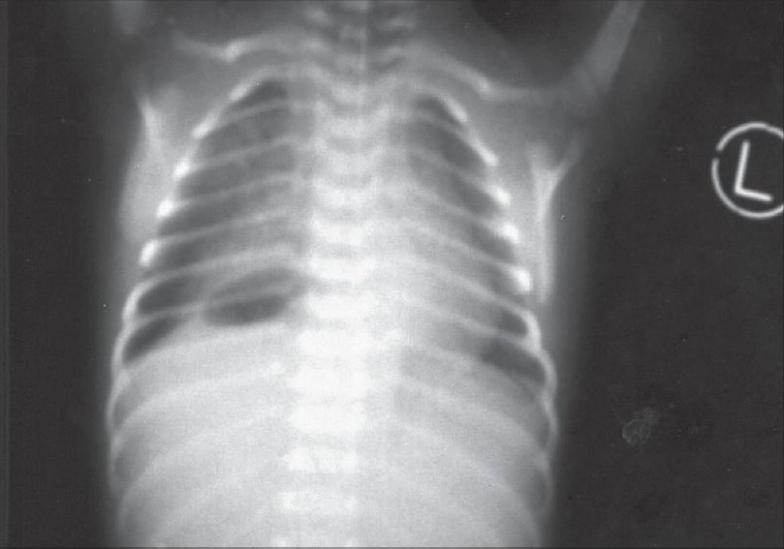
Plane X-ray chest showing the presence of abnormal gas shadow in the right paravertebral region in the lower chest
